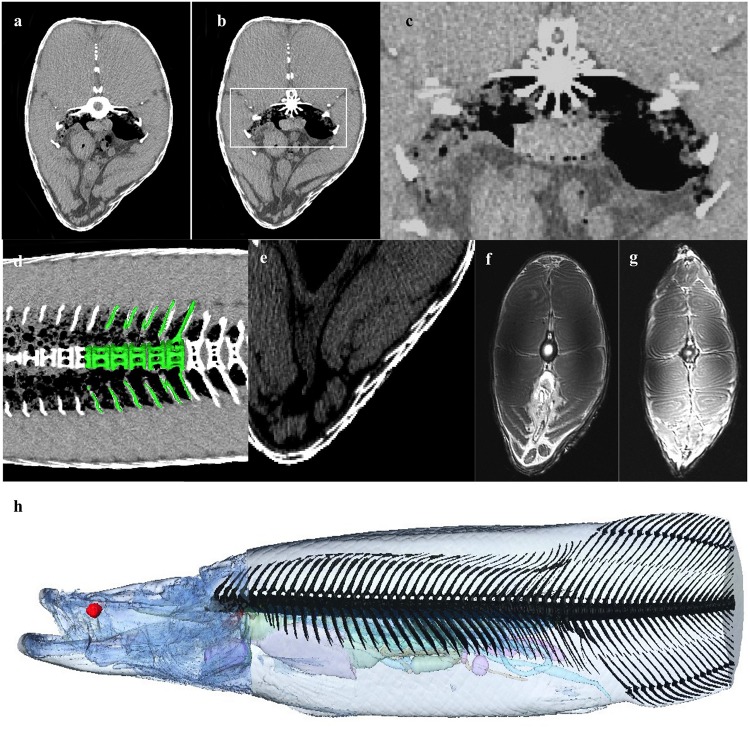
Musculoskeletal and Integument. (a) Axial CT at level of GB demonstrating air partially encased between the bony cortices of the transvers processes of the vertebral bodies, and (b,c) shows spiculation of the vertebral body, possibly to increase surface area of respiratory epithelium exposed to air. (d) 3D rendering of vertebrae on background of coronal CT slice showing air density within part of the in the vertebral body between spicules. (e) Axial CT section demonstrating flexible dermal armor of elasmoid scales made from overlapping layers of type 1 collagen and a highly mineralized hydroxyapatite outer layer (Yang et al., 2014). Scales overlap with a thickness up to 9.7 mm. Single scale thickness is approximately 3.4 mm. (f,g) The muscle is divided in to left and right epaxial and hypaxial muscle by the vertical and horizontal septa. (h) Bony axial skeleton reconstructed from CT data (excluding distal tail).
